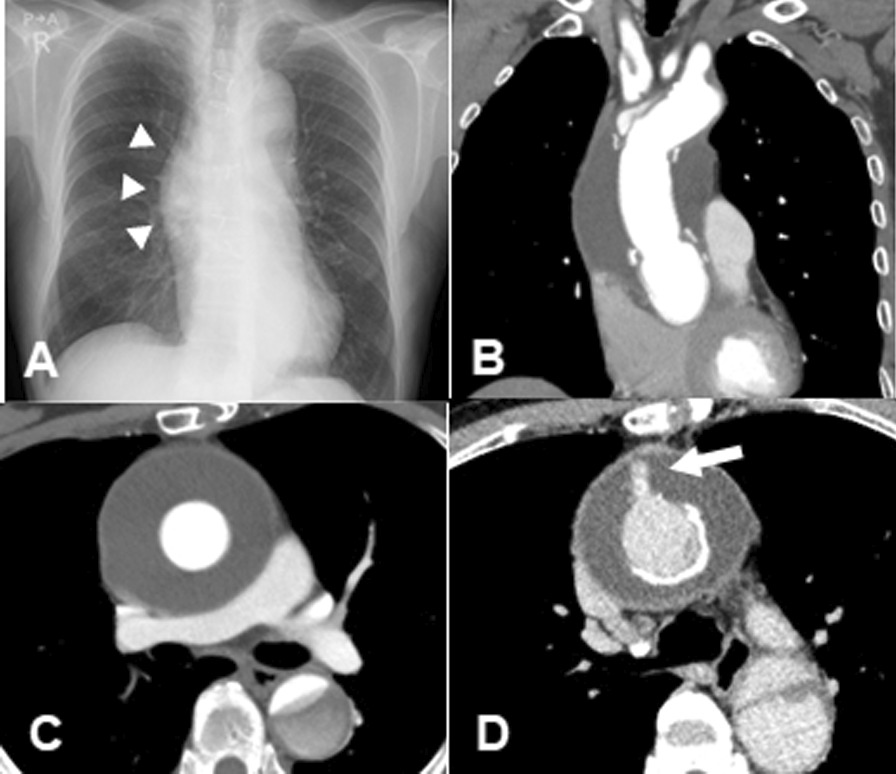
A Chest X-ray revealed the development of a right mediastinal eccentric convex abnormal contour (arrowhead). B, C Computed tomography 1 year after the first surgery showed the expanded large low-density area around the graft (70 × 74 mm). D A contrast effect in the side branch of the graft led to the suspicion of leakage from the stump of the side branch
